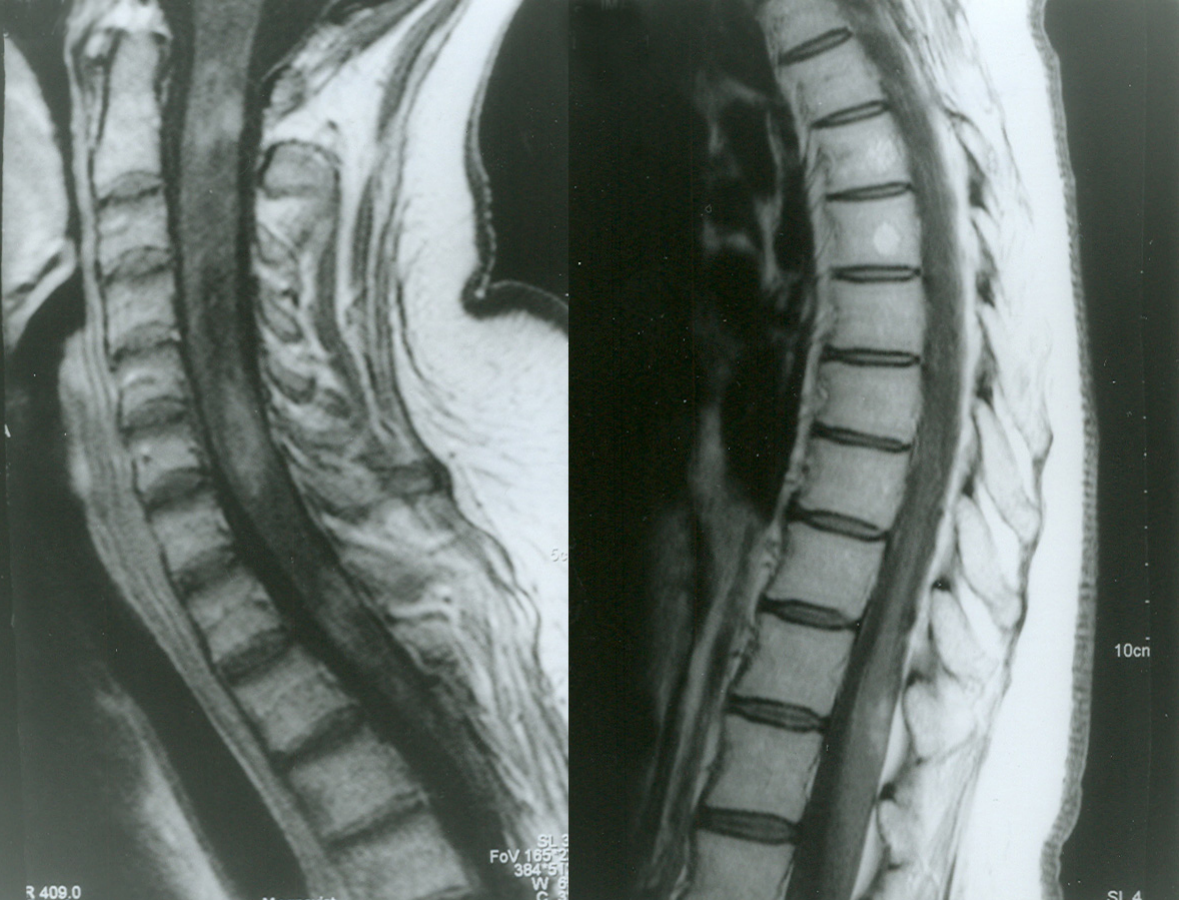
Spinal cord MRI (T1W images with gadolinium) performed in April 2007. MRI shows few areas of pathological gadolinium inhomogenous and ring enhancement, at C2, C4-C5 and Th1 levels. Those changes pointed to aggressive inflammatory changes.
